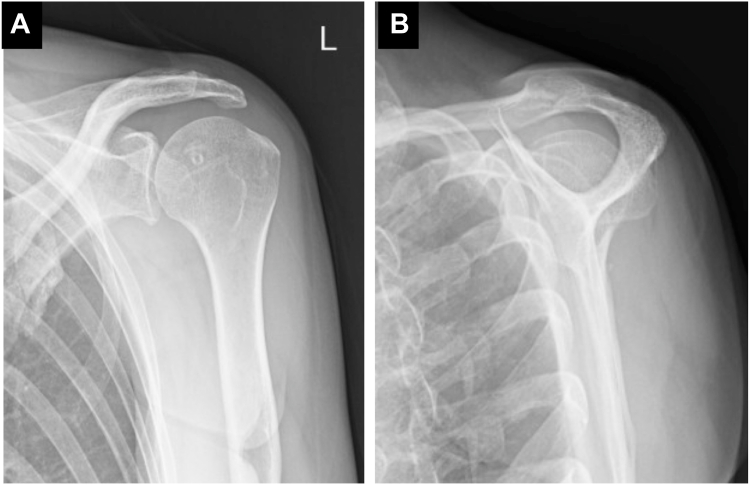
Postoperative radiographs of Case 1. (A) Anteroposterior view. (B) Scapula Y view.
